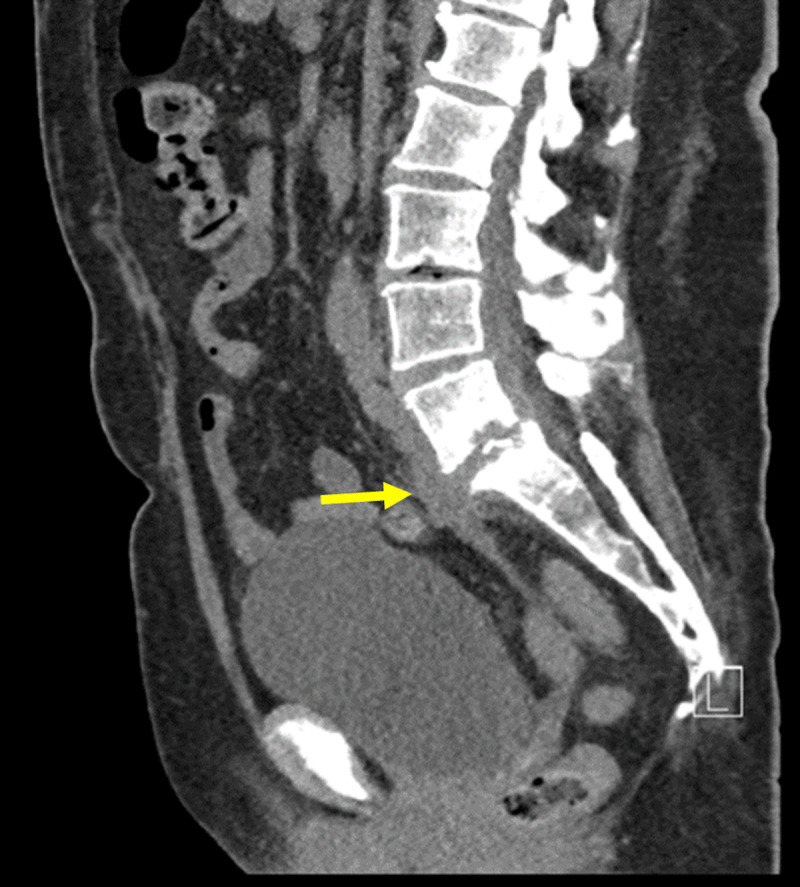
Sagittal contrast CT: Contrast CT-scan showing spondylodiscitis of L5-S1, with infiltration into the soft tissue surrounding S1. Additionally, a 9.7cm fistula can be seen, starting at the intervertebral disk of L5-S1 (yellow arrow) to the vagina.
